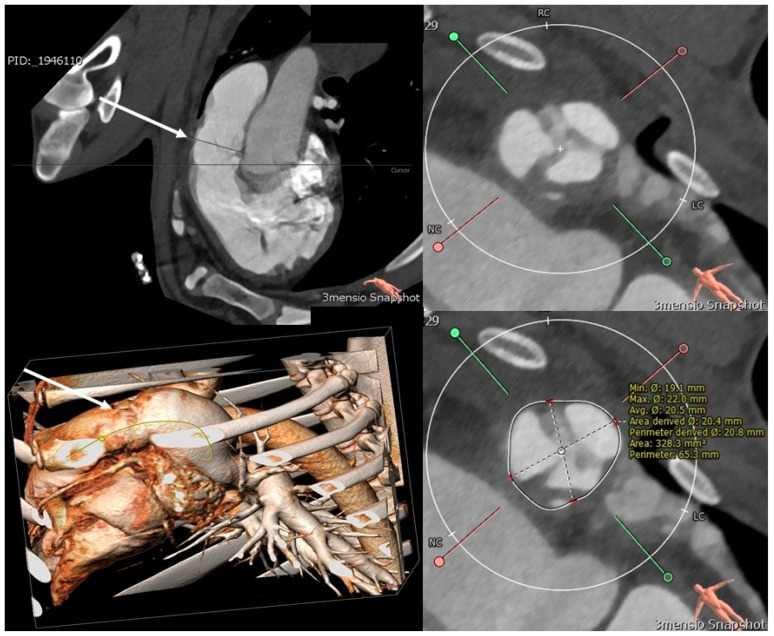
CT scan at time of euthanasia: Upper left image shows the valved conduit in long axis, and lower left image shows a 3D reconstruction of the valved conduit in situ (white arrows). Images on the right side show the conduit valve in short axis with three leaflets (upper image) and measurements (lower image).
